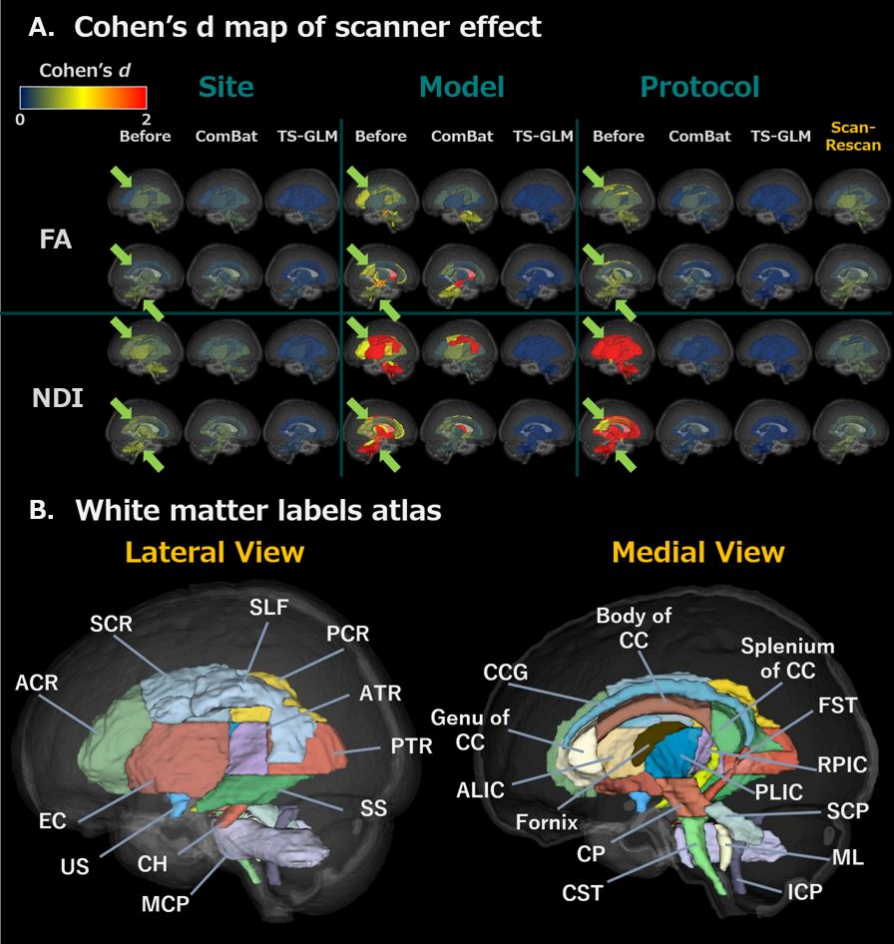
Cohen’s *d* map of scanner effect in brain white matter before and after the harmonization methods. Shown are the maps of scanner effects (Site, MRI models, Protocols) in the WM regions of the standard WM template (JHU ICBM-DTI-81) before and after the harmonization. Color shows effect size evaluated by Cohen’s *d* as the following: blue, *d* = 0; yellow, *d* = 1; and red, *d* = 2. The Cohen’s *d* of scanner effect before harmonization was relatively higher at green arrow. After harmonization using ComBat or TS-GLM, the Cohen’s *d* of scanner effect for both fractional anisotropy (FA) and intra-neurite volume fraction (NDI) decreased in whole WM up to that of scan-rescan in which Cohen’s *d* did not almost change between scan and rescan. However, TS-GLM more largely harmonized scanner effect up to Cohen’s *d* < 0.01 than ComBat. Abbreviations: ACR, anterior corona radiata; CC, corpus callosum; CP, cerebral peduncle; ComBat, combined association test; dMRI, diffusion-weighted magnetic resonance imaging; EC, external capsule; GLM, general linear model; MCP, middle cerebellar peduncle; PCR, posterior corona radiata; SCR, superior corona radiata; TS, traveling subjects; WM, white matter.
